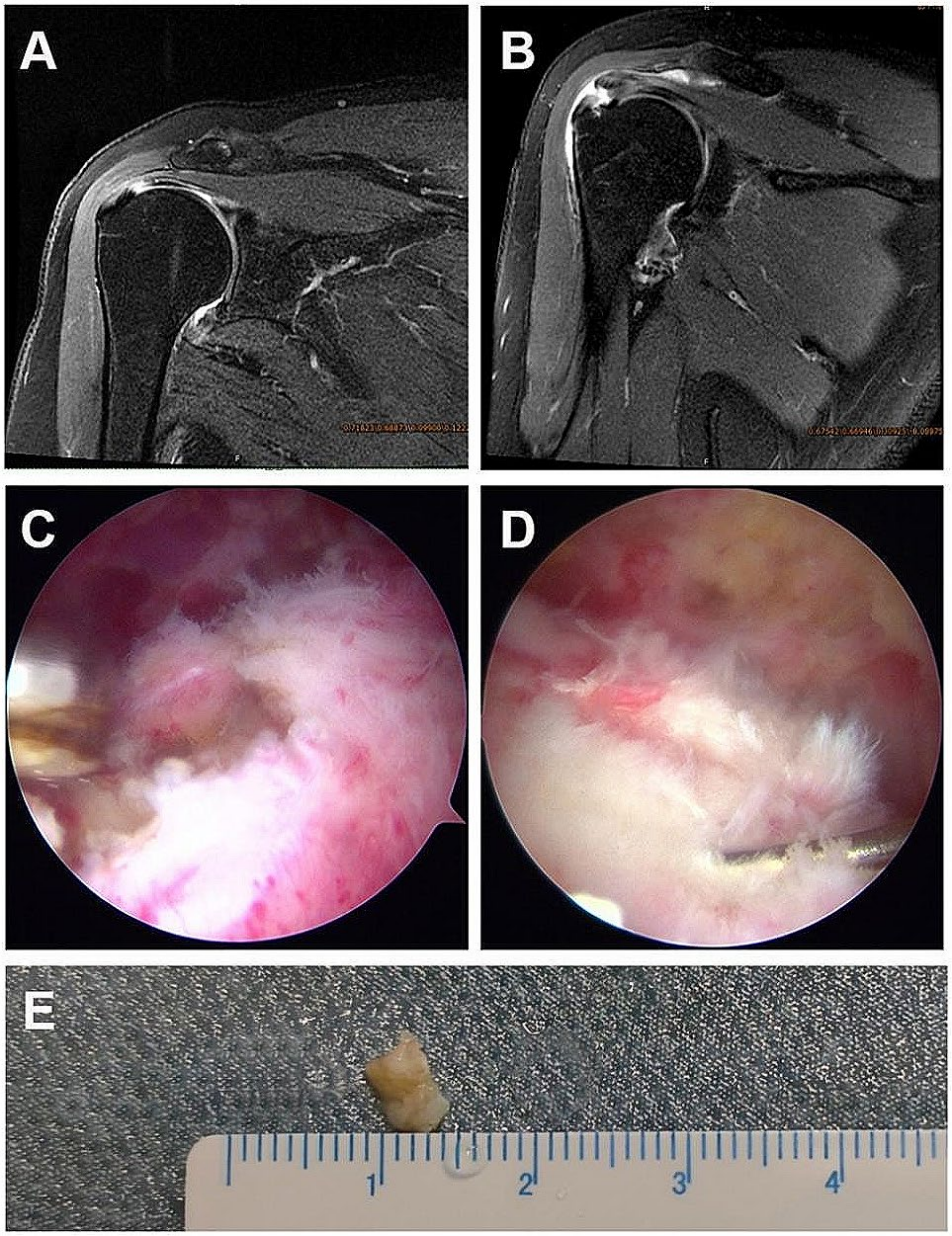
MRI images and arthroscopic view of torn rotator cuff in nondiabetic (A, C) and diabetic patients (B, D). E Macroscopic view of harvested tendon sample
